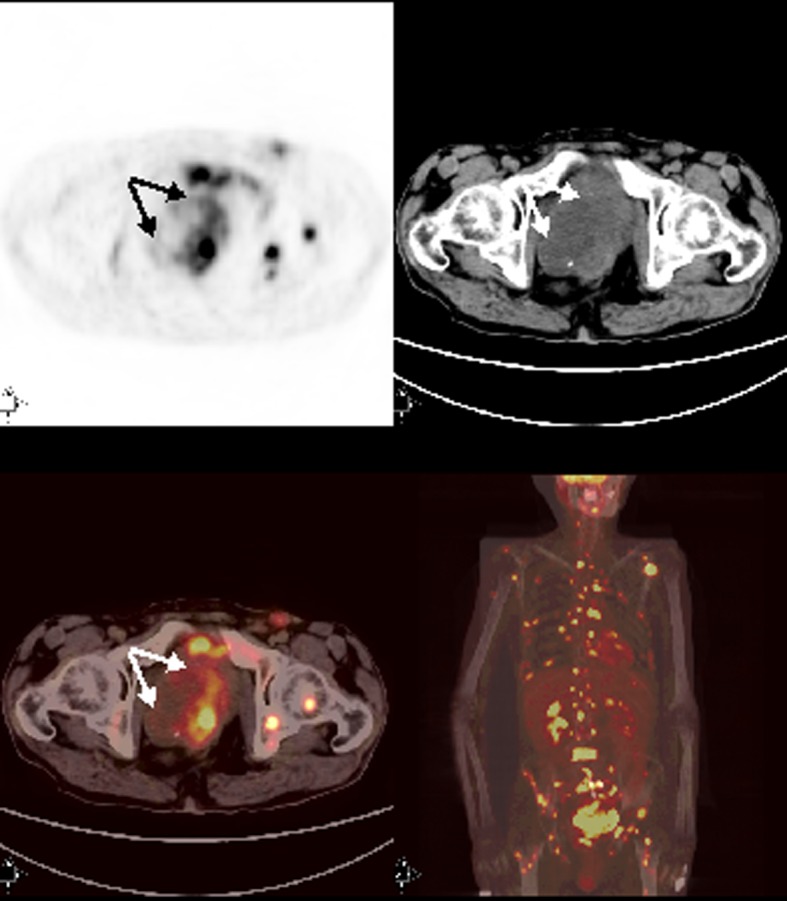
Axial and MIP PET/CT images showed primary tumor with intense FDG accumulation in rectosigmoid. There were also multipl bone-bone marrow metastases and extraskeletal metastases with prominent FDG uptake.
